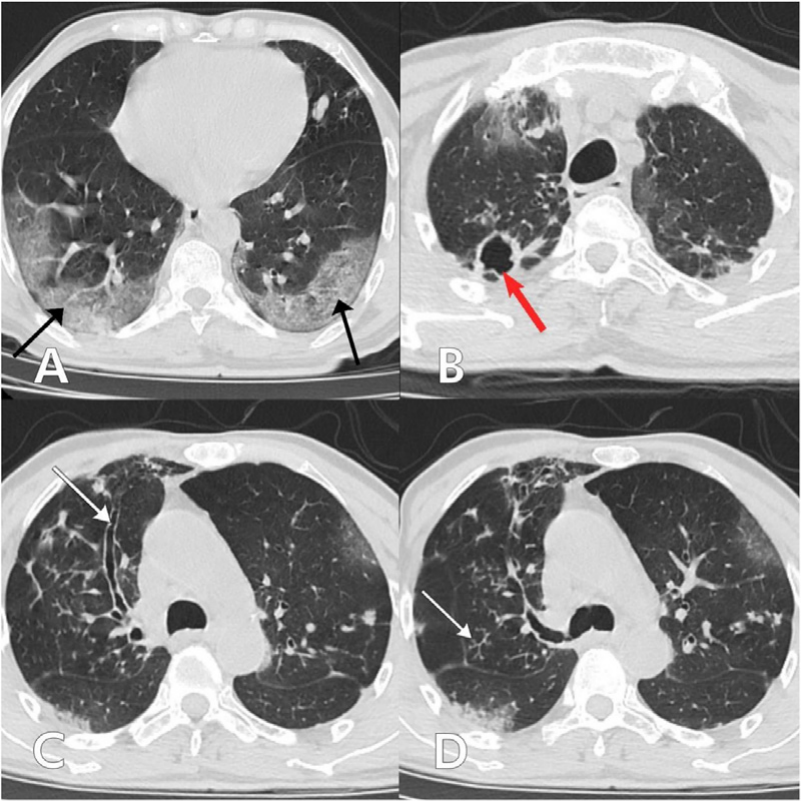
A-D: Axial HRCT thorax showing (A) Peripheral subpleural GGOs (black arrows) with superimposed septal thickening (“crazy paving pattern”) in lower lobes of bilateral lung parenchyma. (B) Cavitatory lesion (red arrow) in right upper lobe with associated fibrotic changes and superimposed ground glass opacities in bilateral upper lobes. (C) Cylindrical bronchiectasis involving right upper lobe (white arrow) with peripheral subpleural GGO in left upper lobe and superior segment of right lower lobe. (D) Centrilobular nodules with “tree-in-bud” appearance in right upper lobe (white arrow) (Color version of figure is available online).
